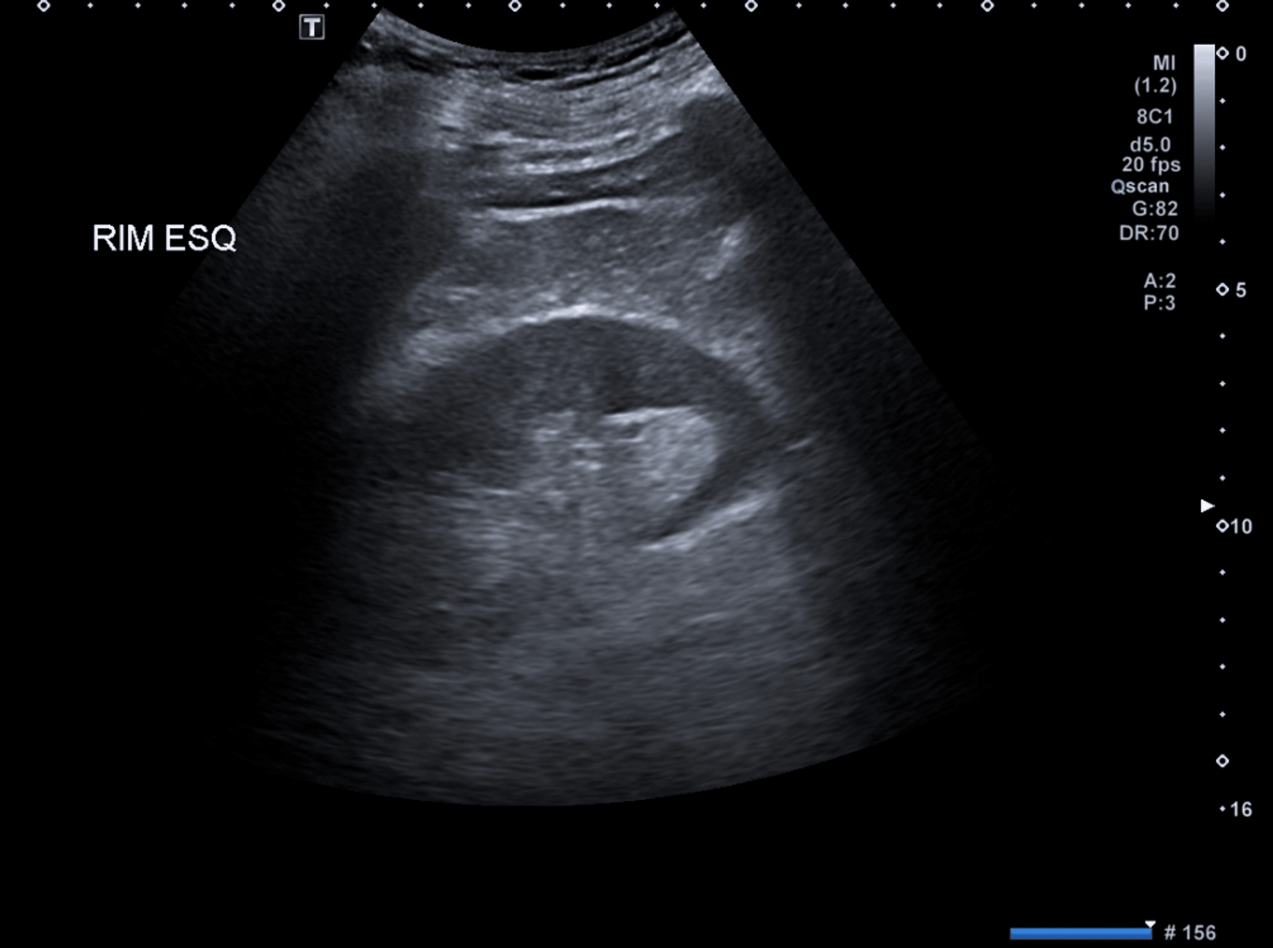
Left kidney ultrasound No changes were observed on this ultrasound.
